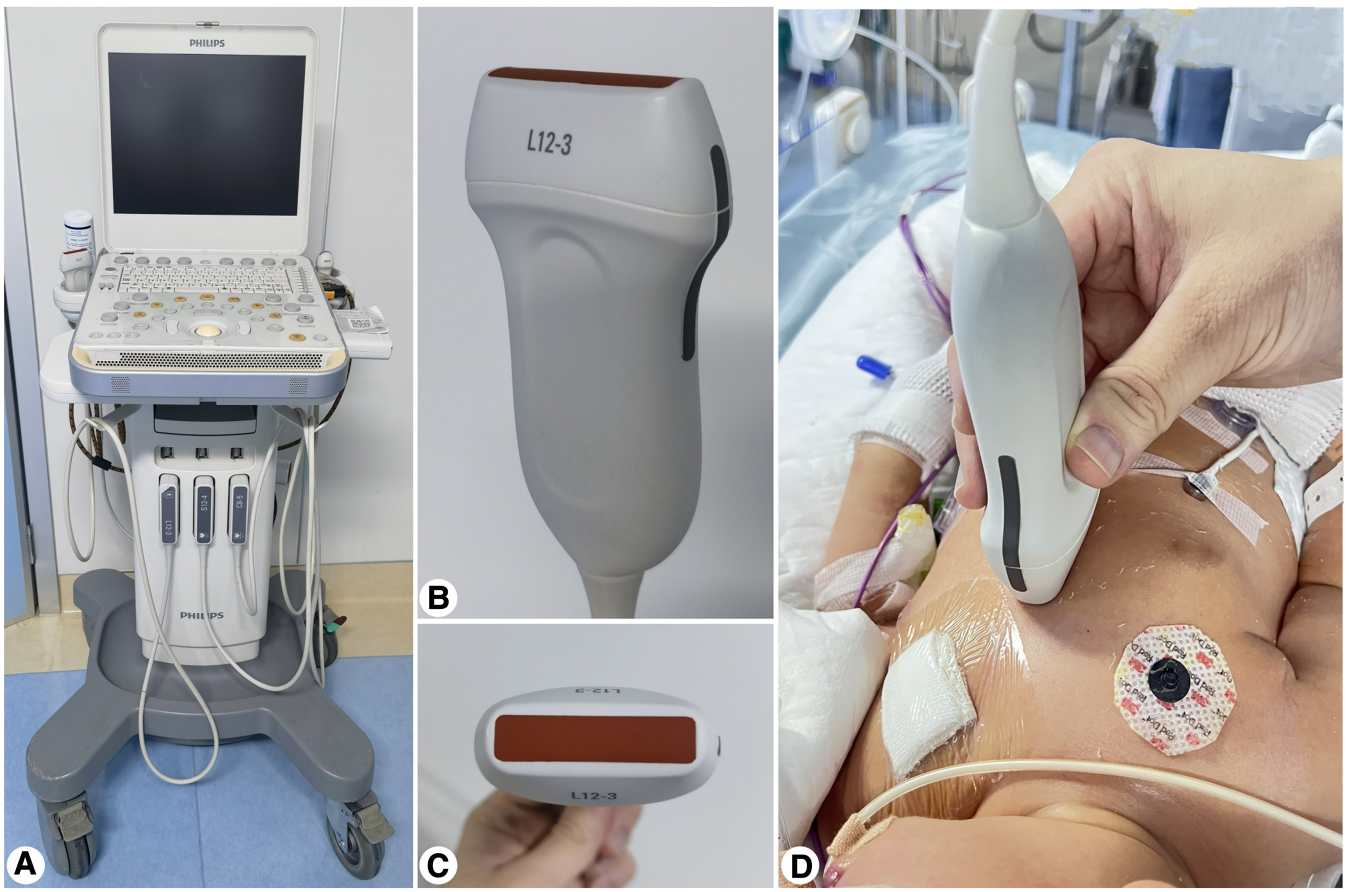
(A) Philips CX50 portable color ultrasound. (B,C) Linear array shallow small organ probe L 12–3 [probe type: broadband linear array probe; frequency range (MHz): 12–3]. (D) Bedside POCUS operation.
